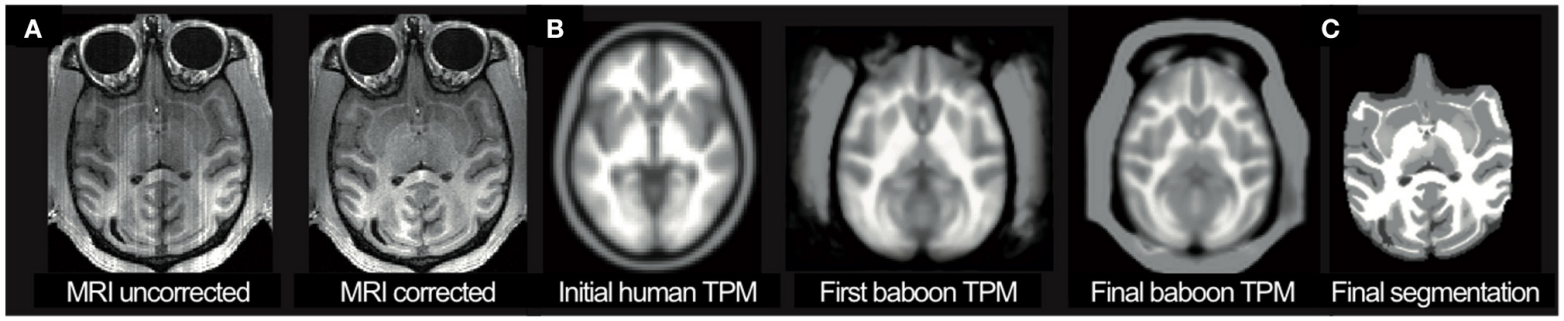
(A) Shown are the original T1-weighted image and the slice-corrected version. (B) For the segmentation process, a baboon specific tissue probability map (TPM; shown as label map) was used in an iterative process, starting with a scaled human template (left) and refinements during each iteration. (C) The final baboon TPM was used to create the final segmentation of the individual MRI.
